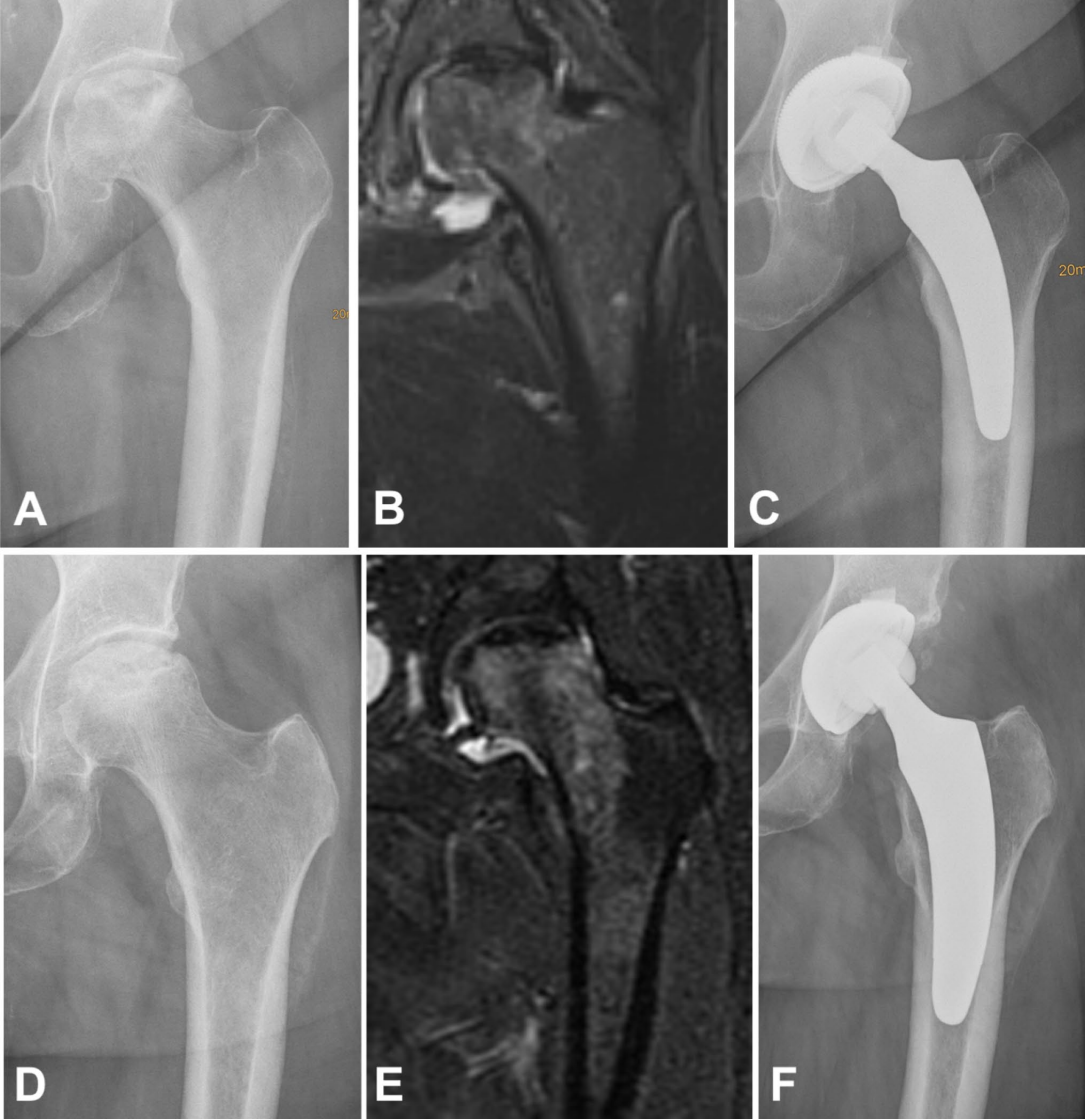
Upper row: if only affecting the femoral head, metaphyseal anchoring based on three-point-anchoring can be aimed for (a preoperative radiograph; b MRI; c postoperative radiograph). Lower row: if also affecting the femoral neck and large parts of the metaphysis, an additional pronounced diaphyseal anchorage should be pursued (d preoperative radiograph, e MRI; f postoperative radiograph)
